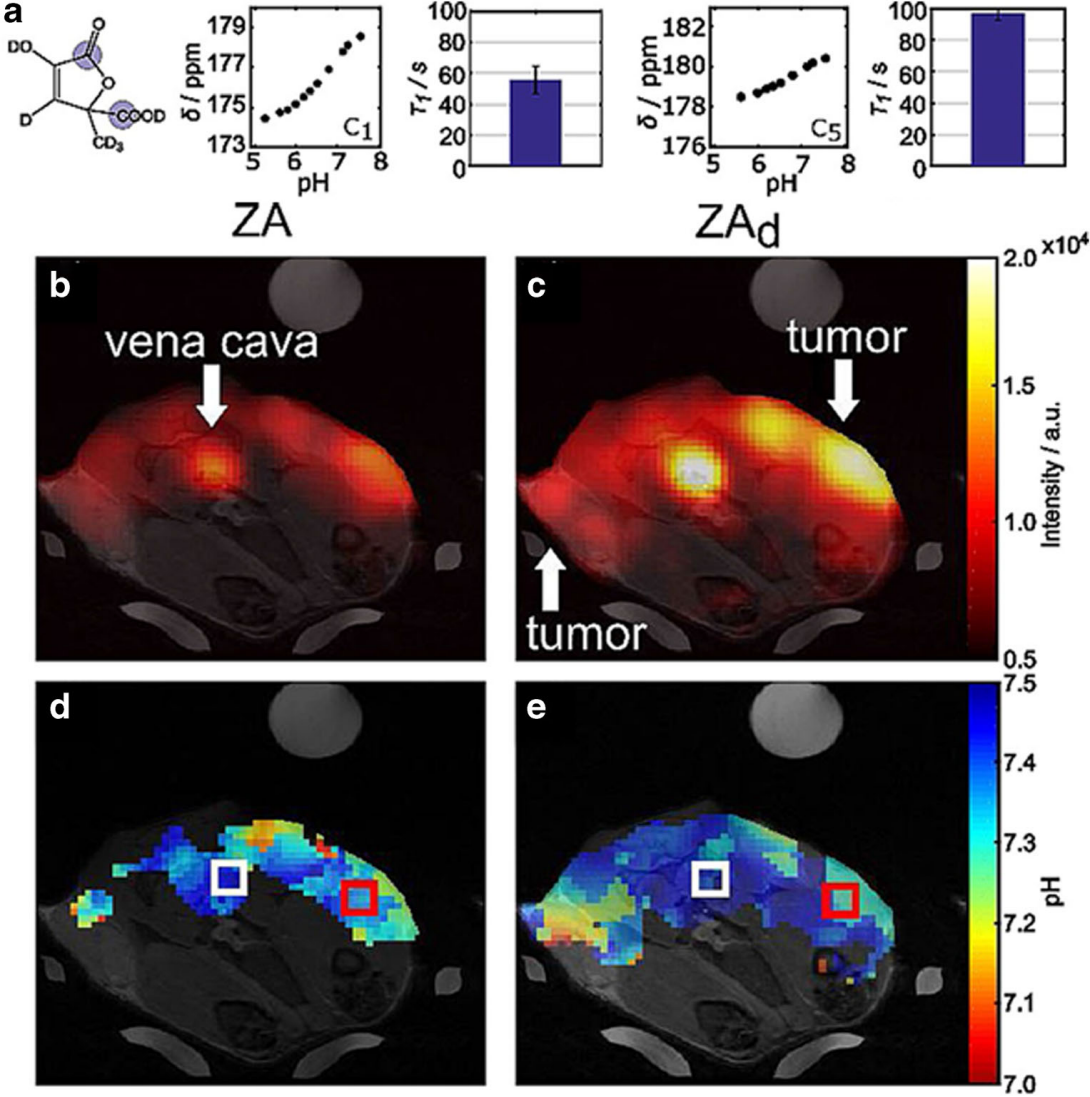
MRI-hyperpolarized imaging of tumor pH. Chemical structure of [1,5-^13^C_2_, 3,6,6,6-D_4_] zymonic acid (ZA_d_) with *T*_1_ values and pH-dependent chemical shifts of the C1 and C5 carbon atoms (a). *In vivo* signal intensity images acquired after tail-vein injection of ZA (b) and ZA_d_ (c) overlaid on anatomical *T*_1_-weighted ^1^H images. pH images calculated from the chemical shift differences of C5 and urea, weighted by the respective signal intensities, show an acidification of the tumor microenvironment for both ZA (d) and ZA_d_ (e). Reproduced with permission from ChemPhysChem 2017, 18, 2422–2425
